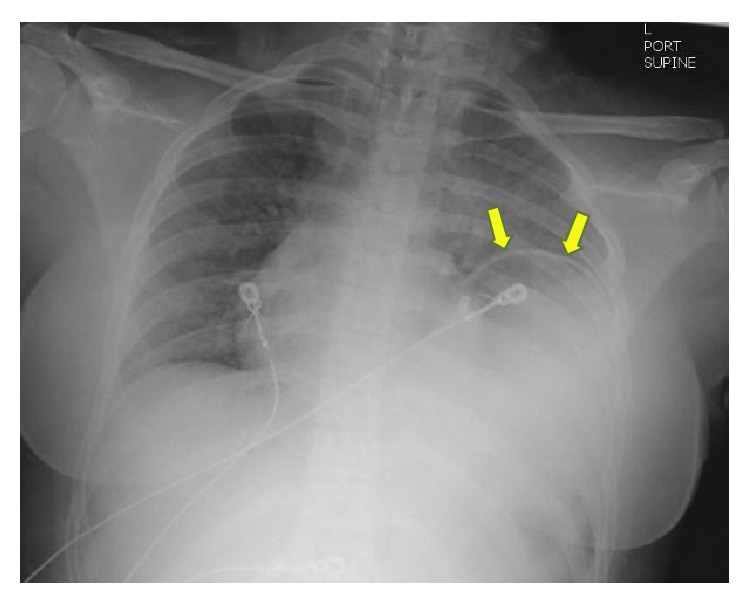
Portable supine chest radiograph shows suspected elevation of the left hemidiaphragm (yellow arrows) with underlying haziness and air-filled viscus over the lower left hemithorax. In retrospect, the suspected “elevated” diaphragmatic contour actually represents the wall of the stomach that herniated through a diaphragmatic hiatus.
